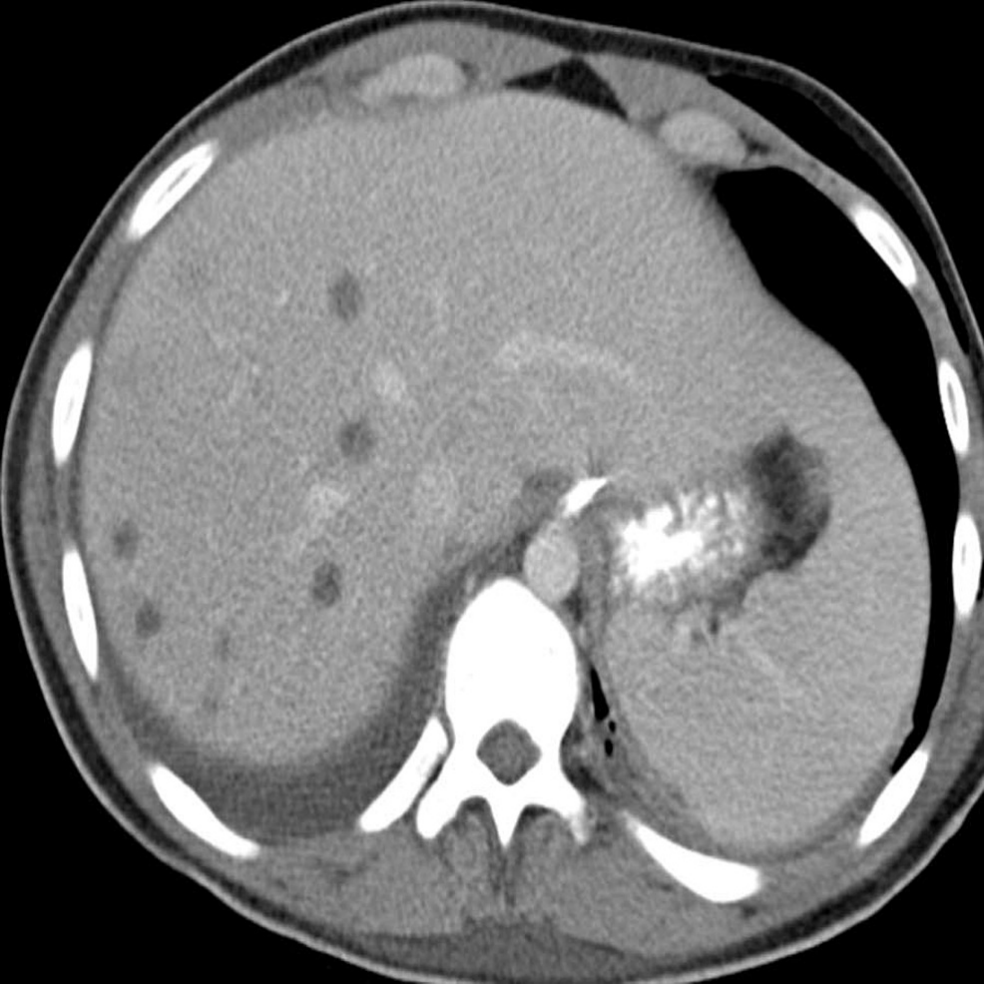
CT abdomen with IV and oral contrast Multiple hypodense lesions within the liver reflect developing septic emboli CT: computed tomography; IV: intravenous
